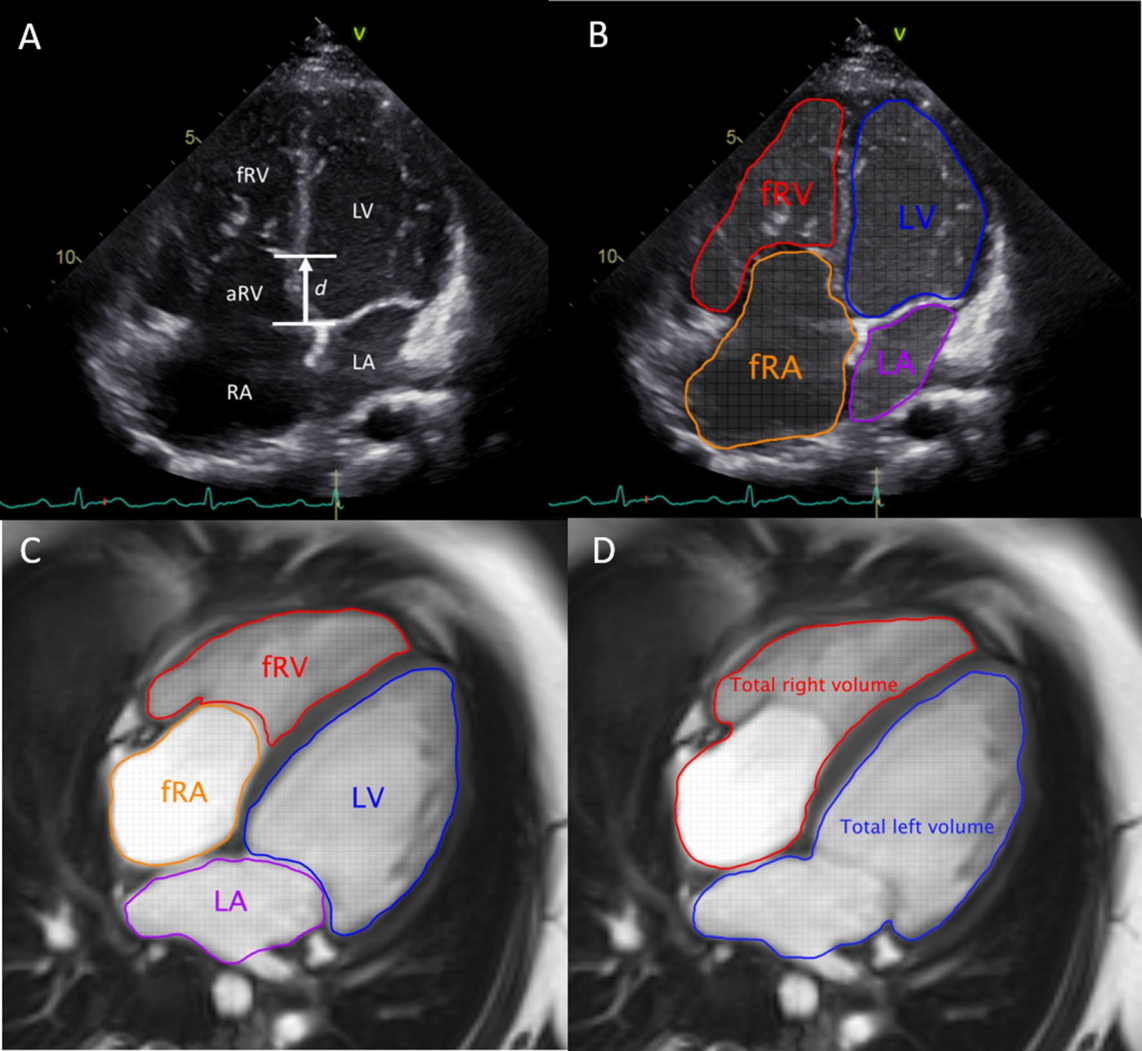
Measurement of the displacement index (A). Celermajer index by echocardiography (B). Overview of segmentation of Celermajer index (C) and total right/left volume ratio (D) on CMR. Note that actual ventricular volumes were obtained on a volumetric stack of short-axis slices. *CMR* cardiac magnetic resonance, *fRA* functional right atrium, *fRV* functional right ventricle, *d* distance, *LA* left atrium, *LV* left ventricle
